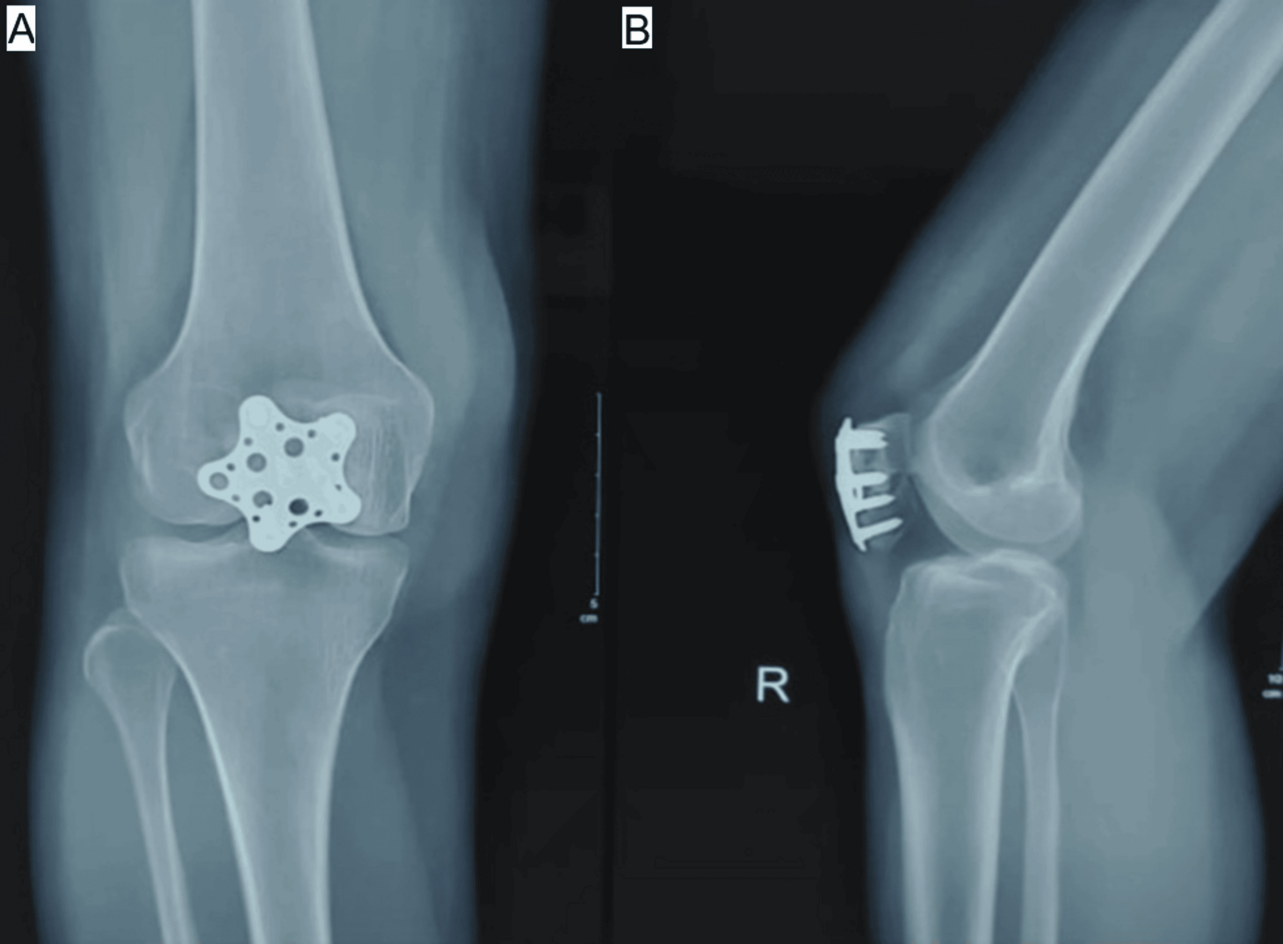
(A, B) Postoperative radiograph of star plate patella fixation in a transverse patella fracture at 6 months. Source: Images courtesy of the authors.
